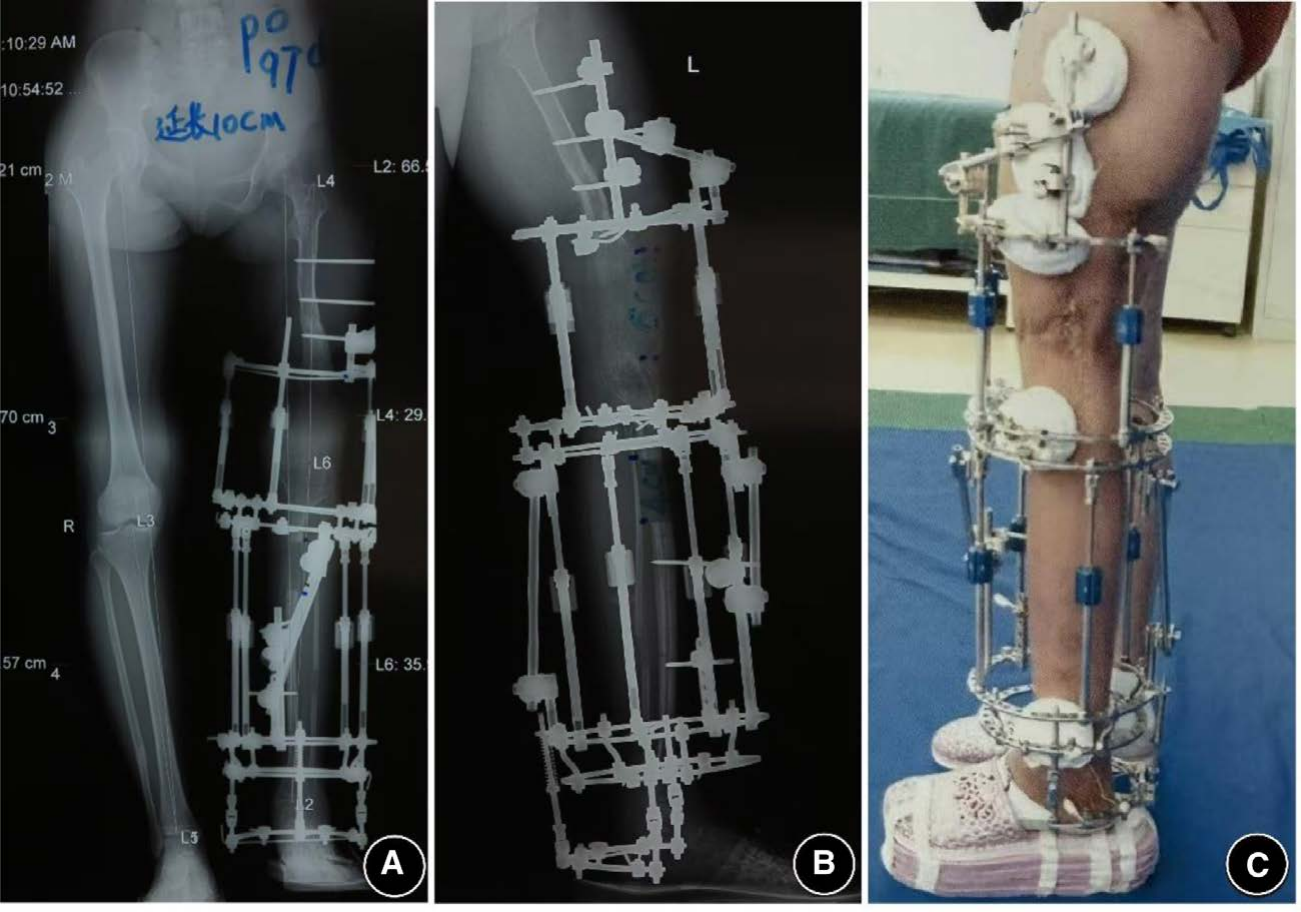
Postoperative day 97 following the second surgery, showing approximately 10 cm of limb lengthening (4 cm tibial lengthening and 6 cm femoral lengthening). (A) Standing anteroposterior radiograph of both lower limbs on postoperative day 97, demonstrating an Ilizarov circular external fixator with bifocal lengthening at the distal femur and proximal tibia of the left side. (B) Standing lateral radiograph of the left lower limb demonstrating ~6 cm femoral lengthening at the distal femoral osteotomy and ~4 cm tibial lengthening at the proximal tibial osteotomy, with columnar regenerate formation at both sites.(C) Lateral weight-bearing clinical photograph of the left lower limb.
